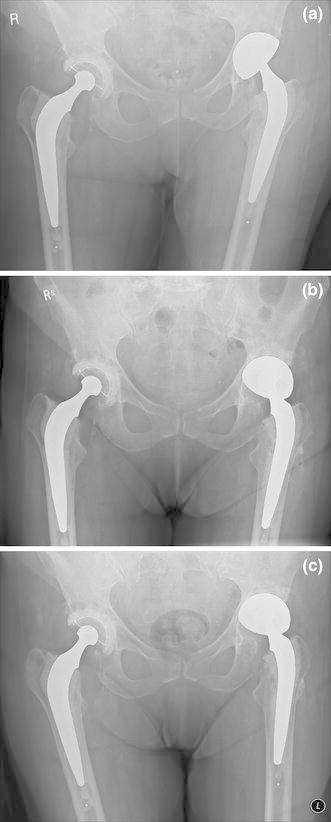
a Immediate postoperative radiograph of an 86-year-old female’s revision THA for recurrent dislocation showing satisfactory socket position. b 6 months postoperative radiographs depicting change in the socket orientation. c Radiograph at 30 months postoperative period with no further change in cup position
